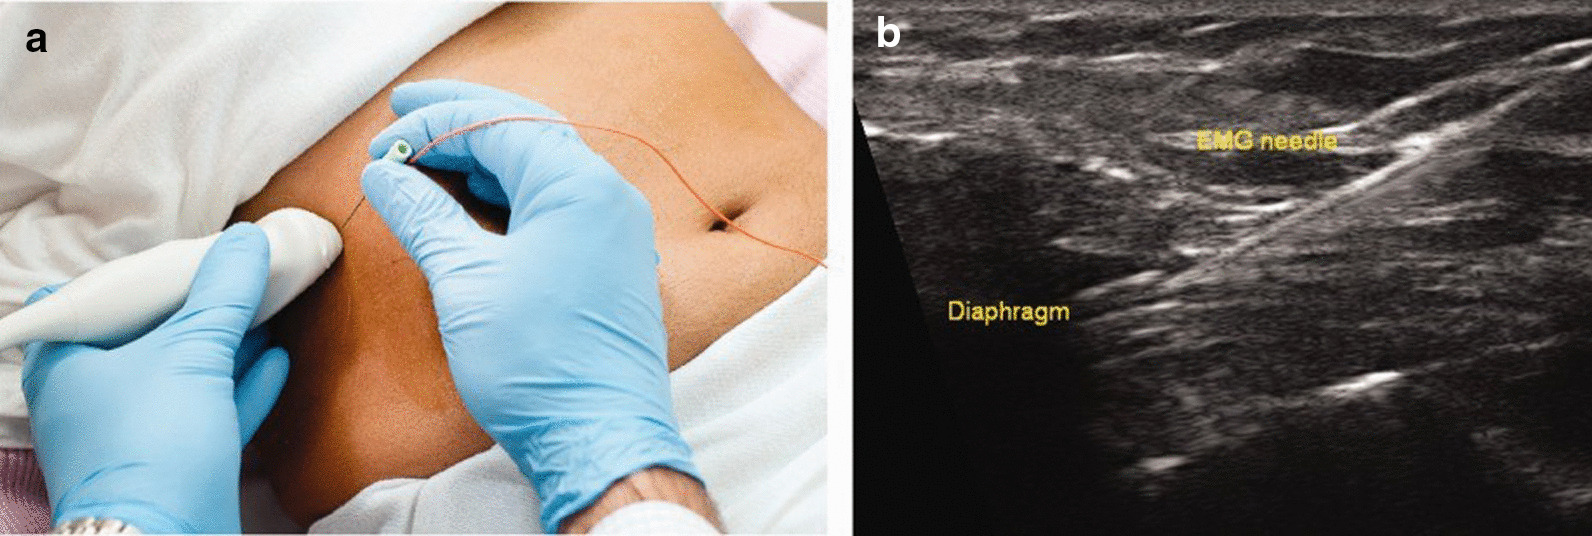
Ultrasound guidance for needle electromyography. (*Left panel, *a) Setup for real‐time ultrasound imaging of the diaphragm during placement of a needle electrode. (*Right panel, *b) Needle electrode penetrating the zone of apposition of the diaphragm. Reproduced with permission from John Wiley and Sons: Sarwal et al. Muscle Nerve 2013;47(3):319–29
